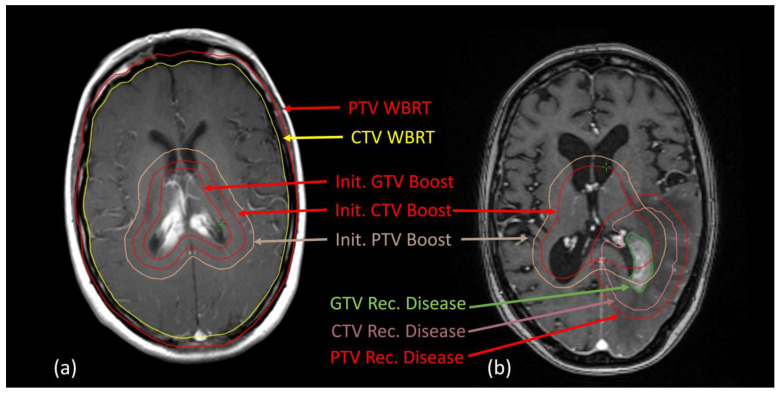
Representative MRI slices matched with RT planning CT. (a) Initial lymphoma manifestation in the area of mid/posterior corpus callosum and corresponding target volumes, (b) local recurrence at the posterior horn of the left lateral ventricle partly overlapping the initial boost volume, 36 months after initial RT treatment. Abbreviations: GTV = gross tumor volume, CTV = clinical target volume, PTV = planning target volume. Treatment was applied via volumetric modulated arc therapy, initial dosing: PTV whole-brain RT in HF volume: 23.4 Gy/1.8 Gy/fraction; PTV Boost: 21.6 Gy/1.8 Gy; PTV recurrent disease: 36 Gy/1.8 Gy/fraction.
